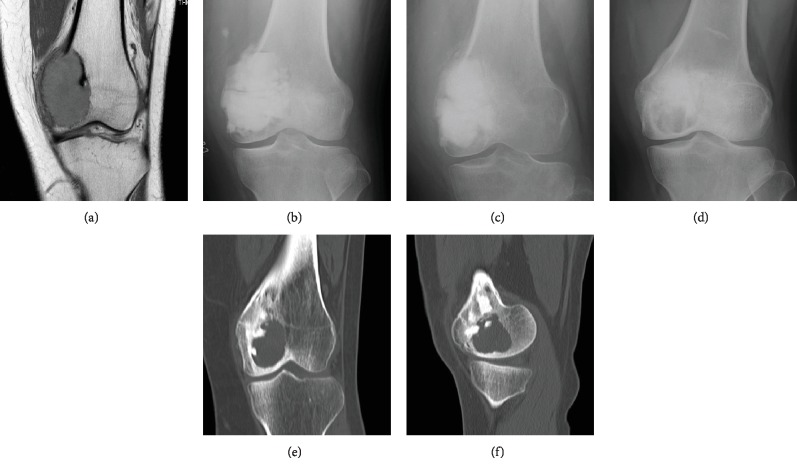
Giant cell tumor of bone in a 25-year-old female. The lesion has homogenous low-signal intensity on T1-weighted images (a). The implanted *β*-TCP is gradually remodeled and incorporated at 3 months (b) and 8 months (c) after the operation. Osteolysis is predominantly observed in *β*-TCP blocks in plain radiographs (d) and a CT image (e) 2 years and 5 months after the operation. The subchondral bone is preserved (f).
